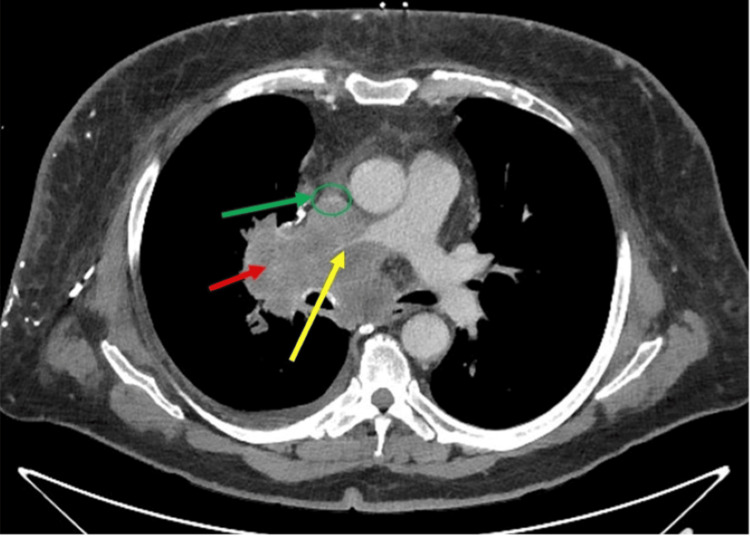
Chest CT scan with contrast (axial section) showing compression of the superior vena cava and right pulmonary artery The mediastinal mass (red arrow) is shown, encasing the superior vena cava (green circle) and right pulmonary artery (yellow arrow)
